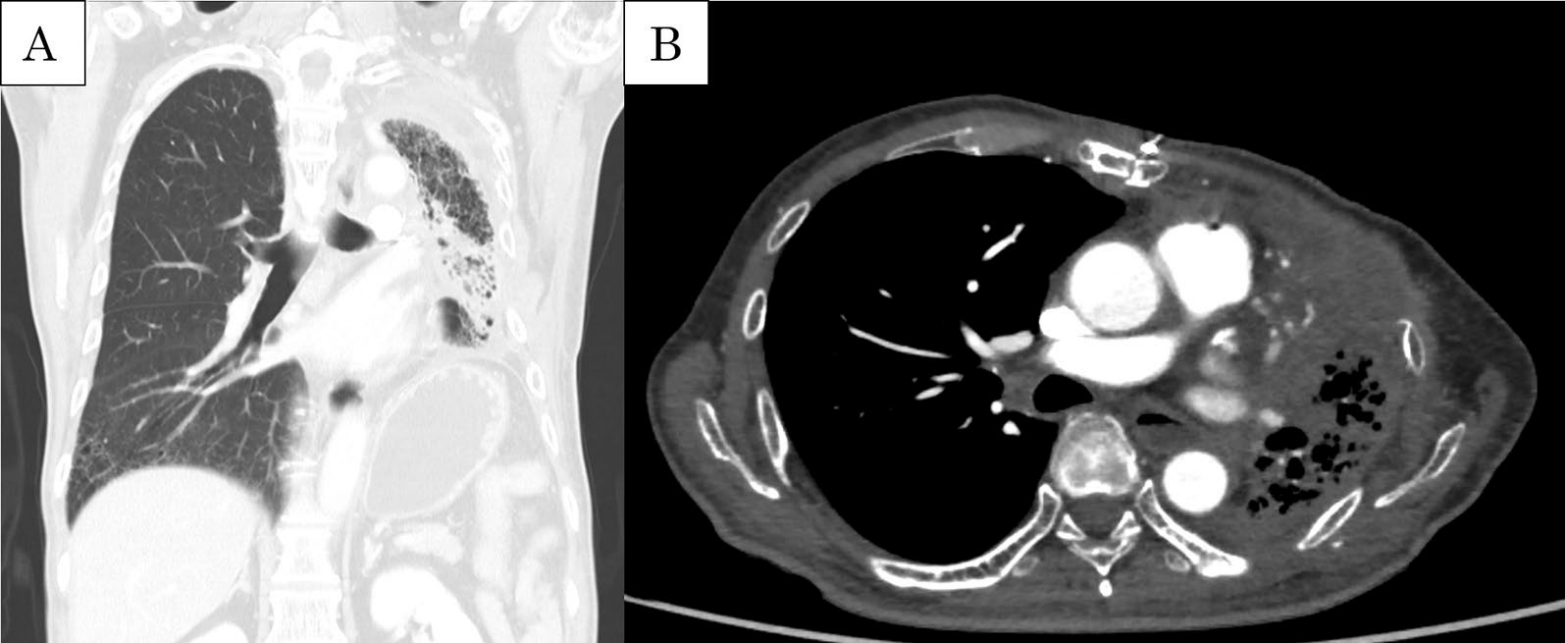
Current CT findings. A Coronal section showing moderate fibrosis in the left lung. B Enhanced CT showing the left pulmonary blood flow supplied by the bronchial artery and collateral circulation from the chest wall
